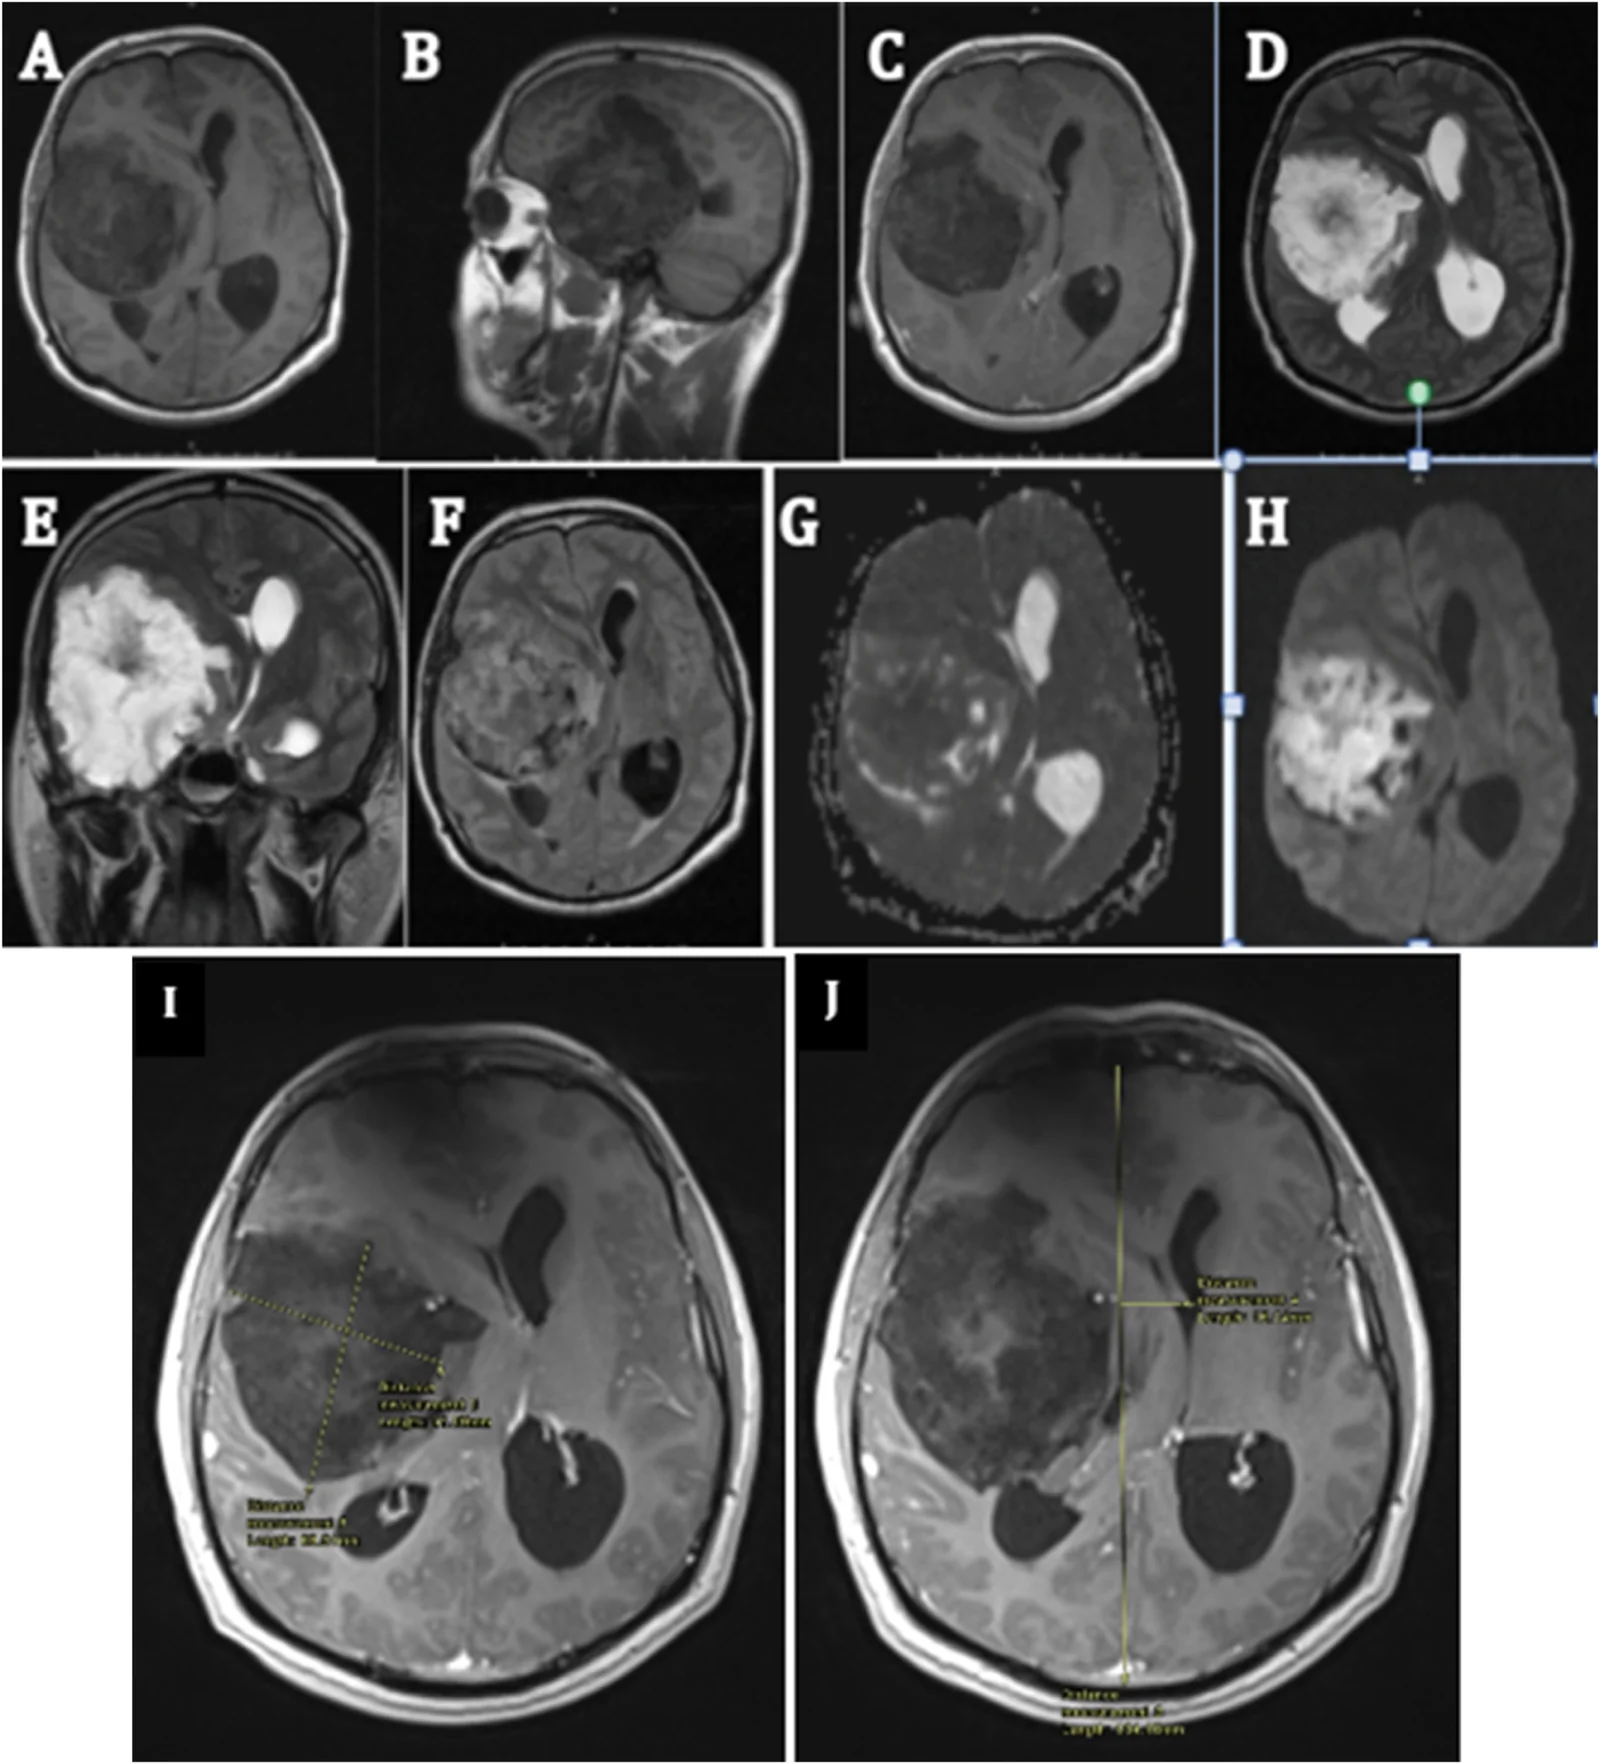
Axial (A) and sagittal (B) T1 images show a low-signal-intensity lesion with a few intrinsic high-signal foci, suggestive of a white epidermoid. Axial T1 post-gadolinium image shows no evidence of enhancement (C). Axial (D) and coronal (E) T2 images show a hyperintense lesion. Axial FLAIR image shows heterogeneous/dirty signal (F). Axial diffusion (G) and ADC (H) images show restricted diffusion. The size of the cyst (I) and the extent of midline shift (J) are depicted in axial sections.
